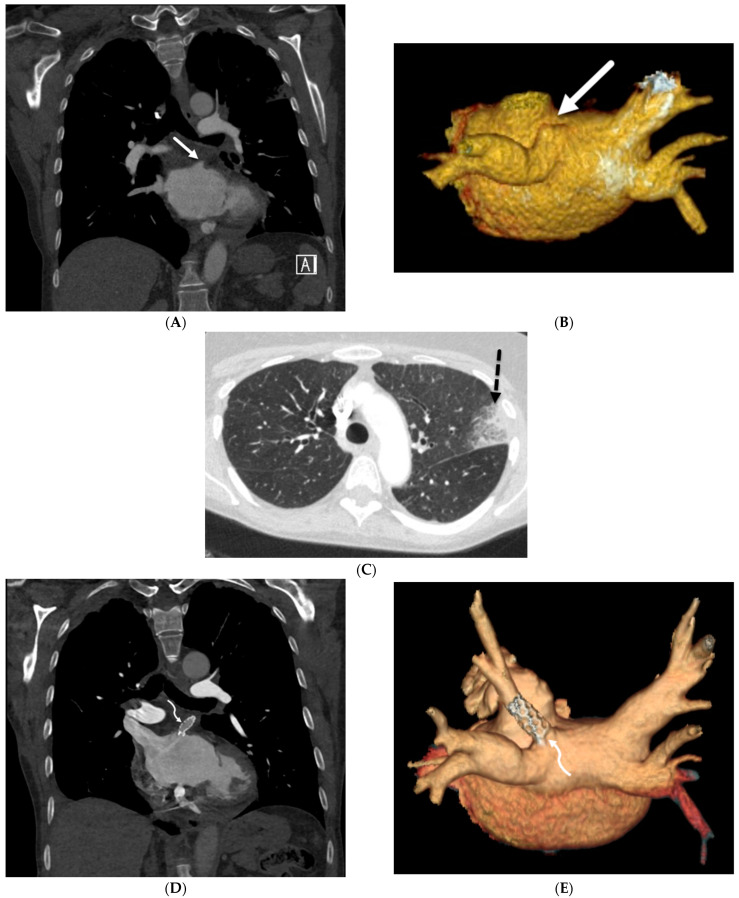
A 73-year-old female with atrial fibrillation refractory to medication post catheter ablation. Coronal chest CT and posterior view volume-rendered images of left atrium and pulmonary veins (A,B) demonstrate abrupt cut off of the left superior pulmonary vein (solid white arrow). An axial chest CT image (C) shows focal ground glass opacity and interlobular septal thickening (dashed black arrow) in the upper left lobe, representing pulmonary venous congestion and developing pulmonary venous infarct. Subsequent coronal chest CT (D) and posterior view volume-rendered images of the left atrium and pulmonary veins after stent placement (E) demonstrate the patency of the left superior pulmonary vein (curved white arrow).
